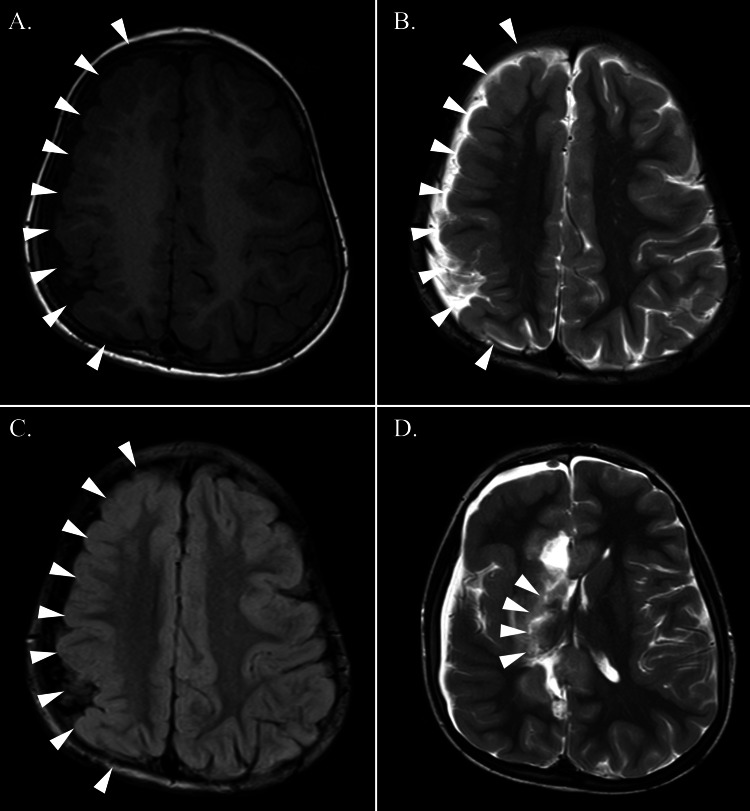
Pre-surgical and post-surgical MRI findings. Pre-surgical T1-weighted MRI (A), T2-weighted MRI (B), and FLAIR imaging (C) at four years of age. MRI shows diffuse right hemisphere polymicrogyria and atrophy (arrowhead), and normal findings in the left hemisphere. (D) Post-surgical T2-weighted MRI shows a disconnection line (arrowhead). FLAIR: fluid-attenuated inversion recovery
